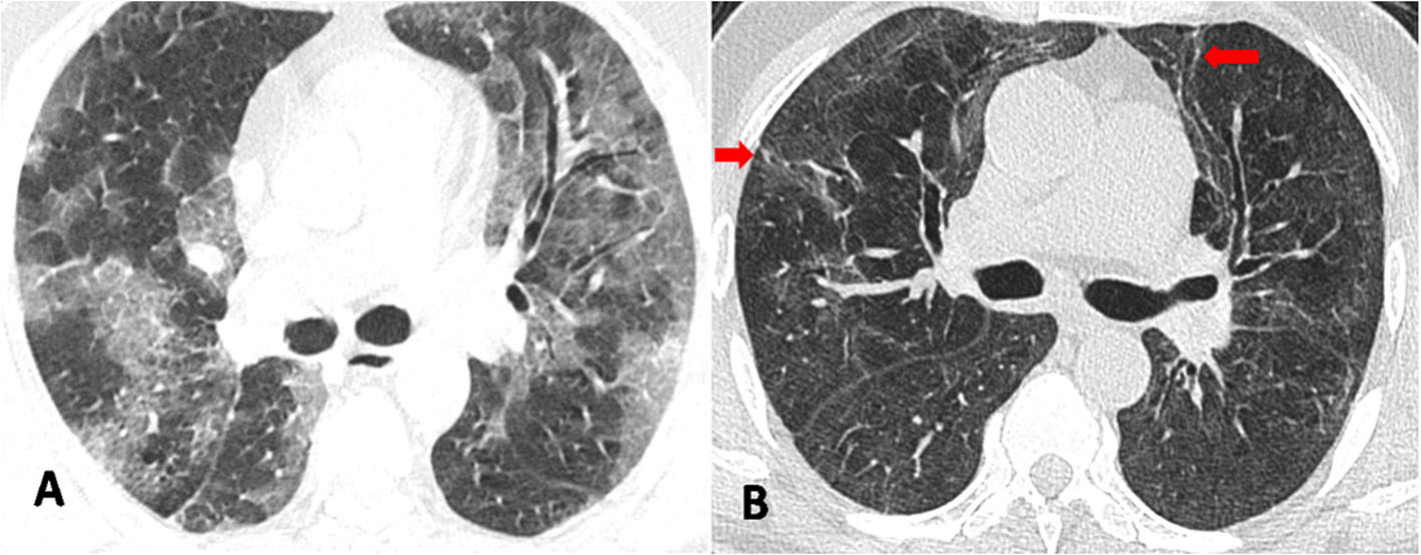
Axial chest CT image in lung window settings of a 52-year-old COVID-19 patient showing diffuse ground glass opacification in both lungs just below the level of the carina at the time of hospital admission (a). Follow-up CT performed 97 days after the first CT reveals residual faint ground glass opacities in both lungs with architectural distortion and bronchiectasis (red arrows) (b)
